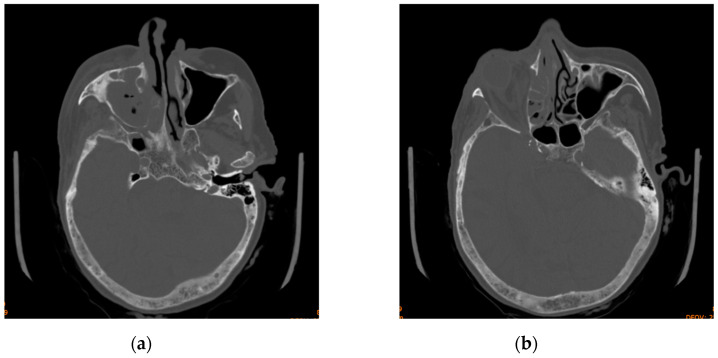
Axial section of computed tomography scan displaying (a) maxillary sinus opacification and (b) ethmoid sinus opacification.
